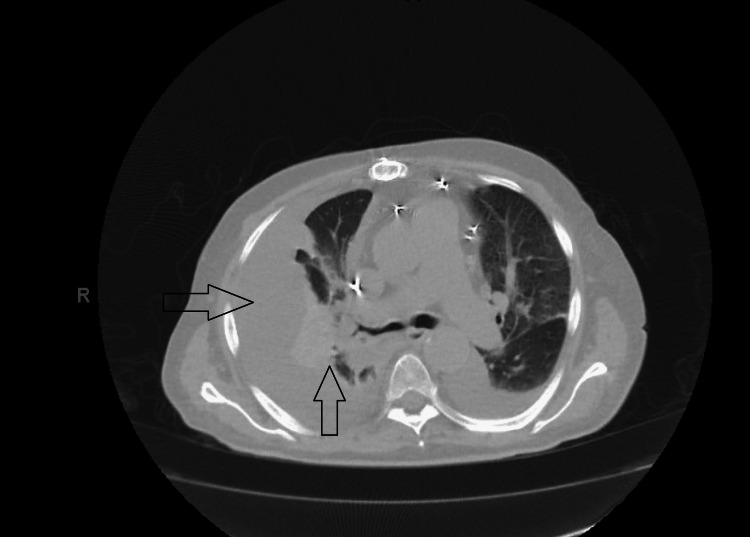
CT scan of the chest showing right lower lobe pneumonia with empyema (arrows) CT: computed tomography
